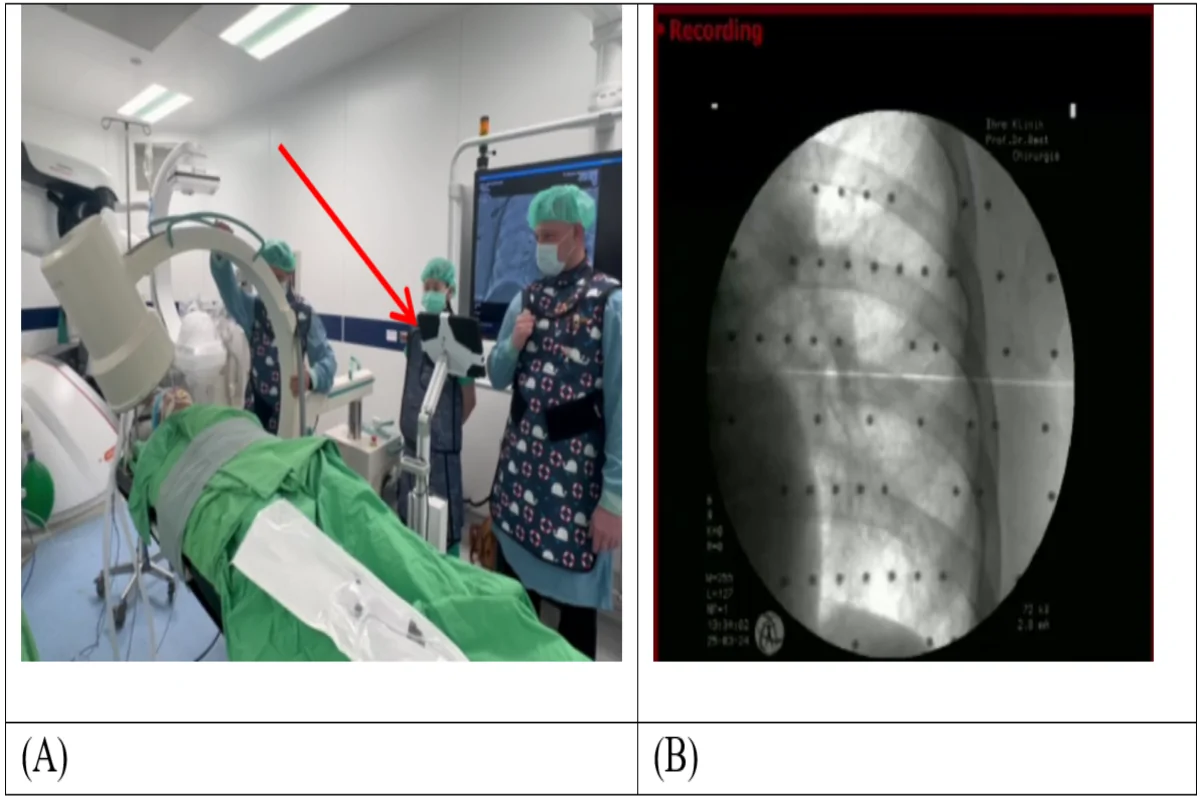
Augmented fluoroscopic localization using the LungVision system. (A) The patient is positioned on a dedicated reference board embedded with radiopaque markers. The LungVision main unit processes intraoperative computed tomography (CT) images acquired by the C-arm and displays navigation guidance via the tablet interface (red arrow). (B) During C-arm rotation, real-time imaging captures a sequence focused on the lesion, which is visualized on the tablet. This AI-enhanced system reconstructs 3D anatomy from standard 2D fluoroscopy to support intraoperative localization by reducing the impact of CT-to-body divergence.
